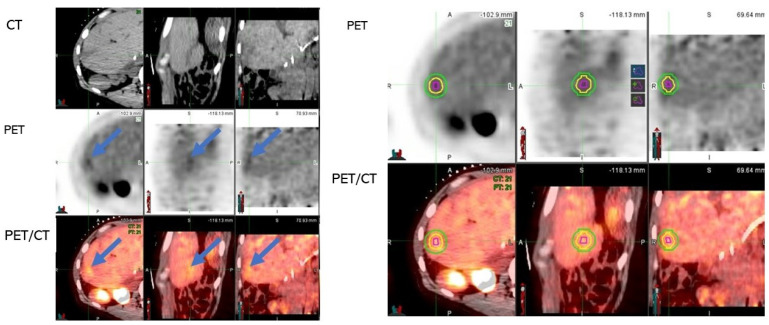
An example of a CLM with low-FDG avidity and 5− and 10−mm margins assessment for the target CLM.
